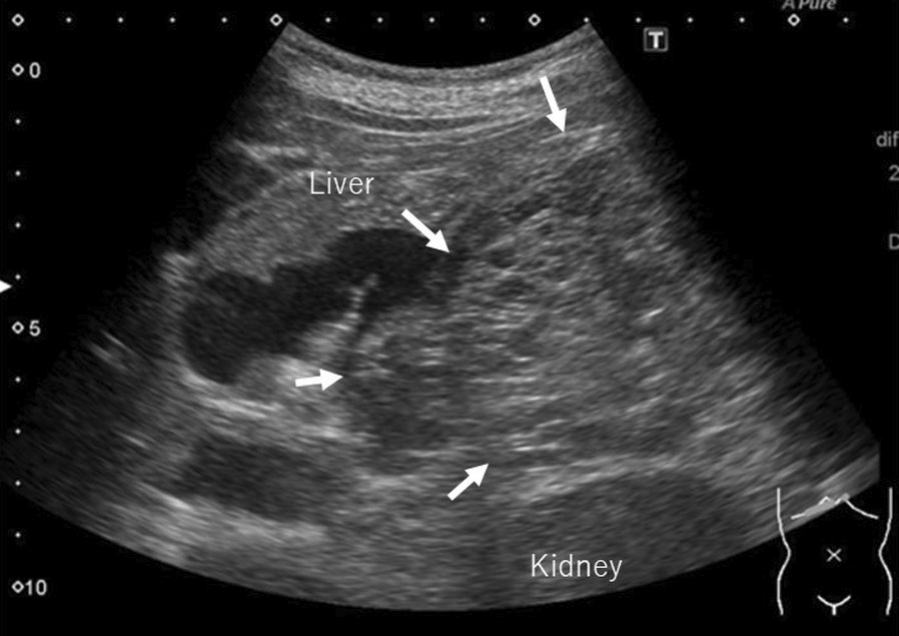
The large irregular mass lesion with a well-defined margin (arrow) compressing surrounding intraabdominal and retroperitoneal organs was seen on extracorporeal ultrasonography. The tumor content showed multi-cystic lesions
